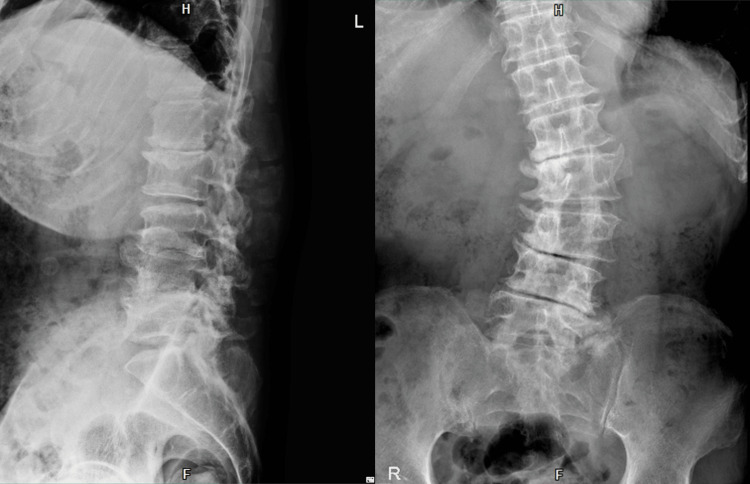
Lumbar spine radiograph at the admission
